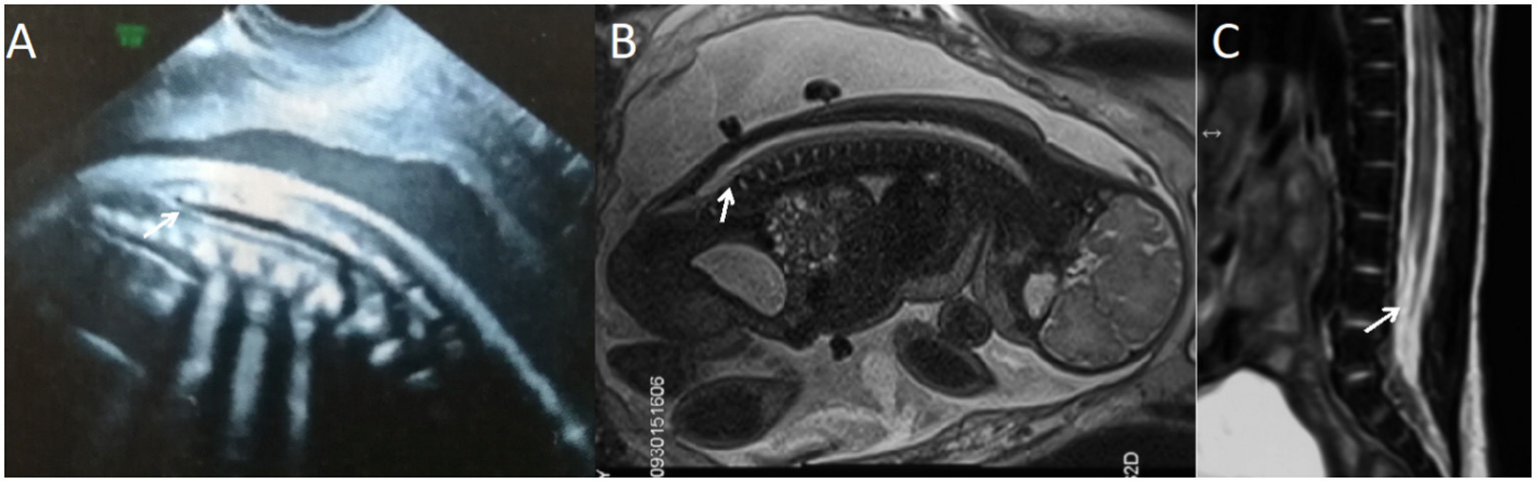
A fetus with simple spinal tethered cord syndrome. (A) The prenatal ultrasound examination at 20 + 5 weeks of gestation showed the lower conus medullaris. (B) Fetal magnetic resonance imaging (MRI) examination at 20 + 6 weeks of gestation showed the lower conus medullaris. (C) T2 sagittal MRI of the lumbosacral vertebrae 22 days after birth suggested simple spinal tethered cord syndrome.
